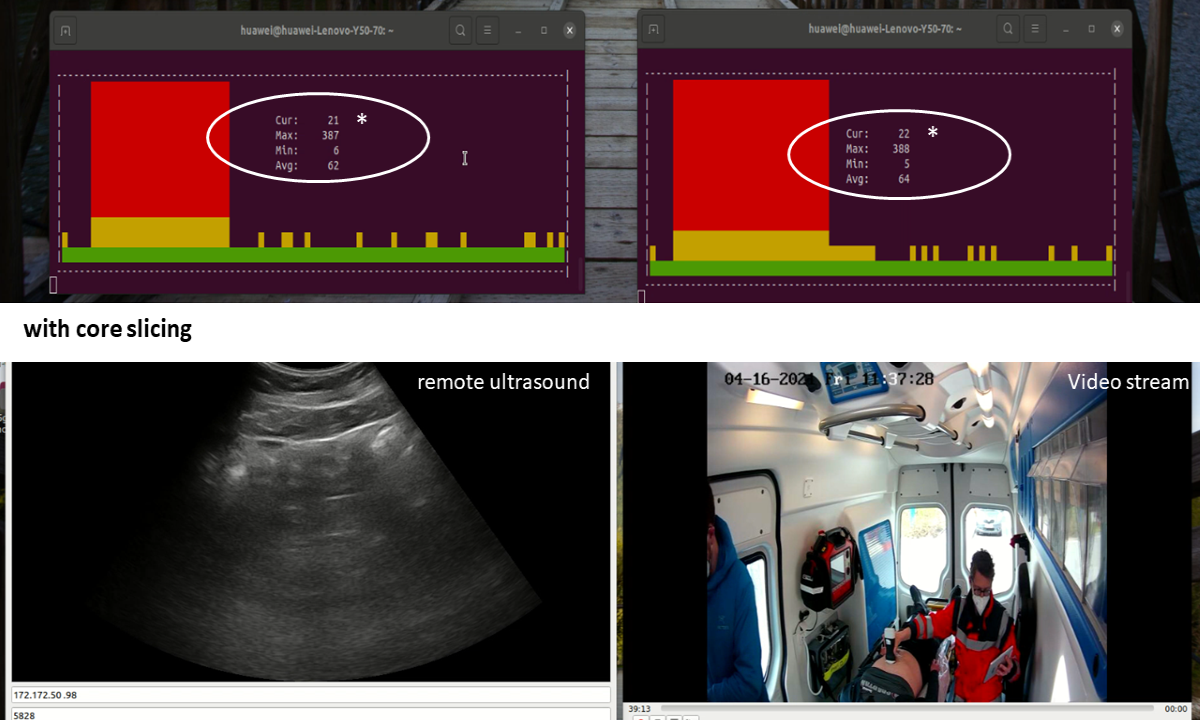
Additional uplink traffic (end-to-end round trip latency *) with core slicing. Ultrasound application (left) and video streaming (right).
